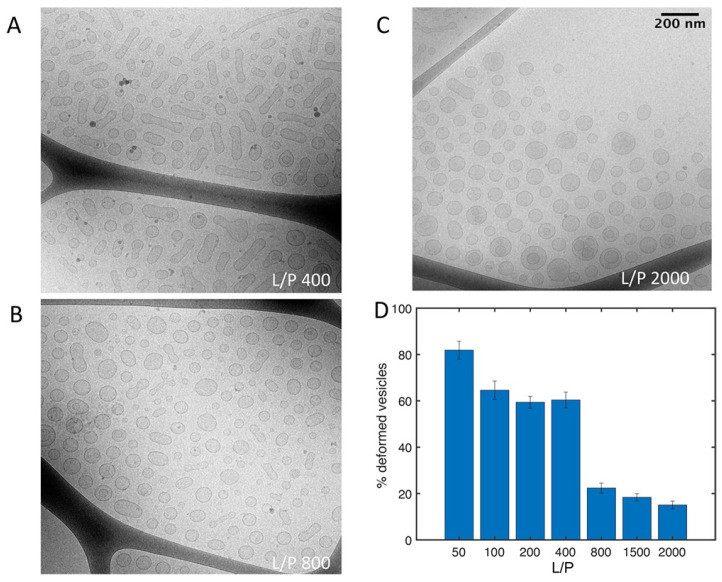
α-Synuclein binding to 70 mol% POPS–30 mol% DOPS SUVs studied with cryo-TEM. (A−C) Examples of cryo-TEM images of small unilamellar vesicles (SUVs) with α-synuclein at the indicated lipid/protein ratios. The scale bar is the same as in (C) for all images. (D) Percentage of deformed vesicles (means ± SD) calculated from six different images of each sample from one experiment assuming no fusion of vesicles. The number of analyzed vesicles was 5330. The figure was taken with permission from ref. [107] (open source).
